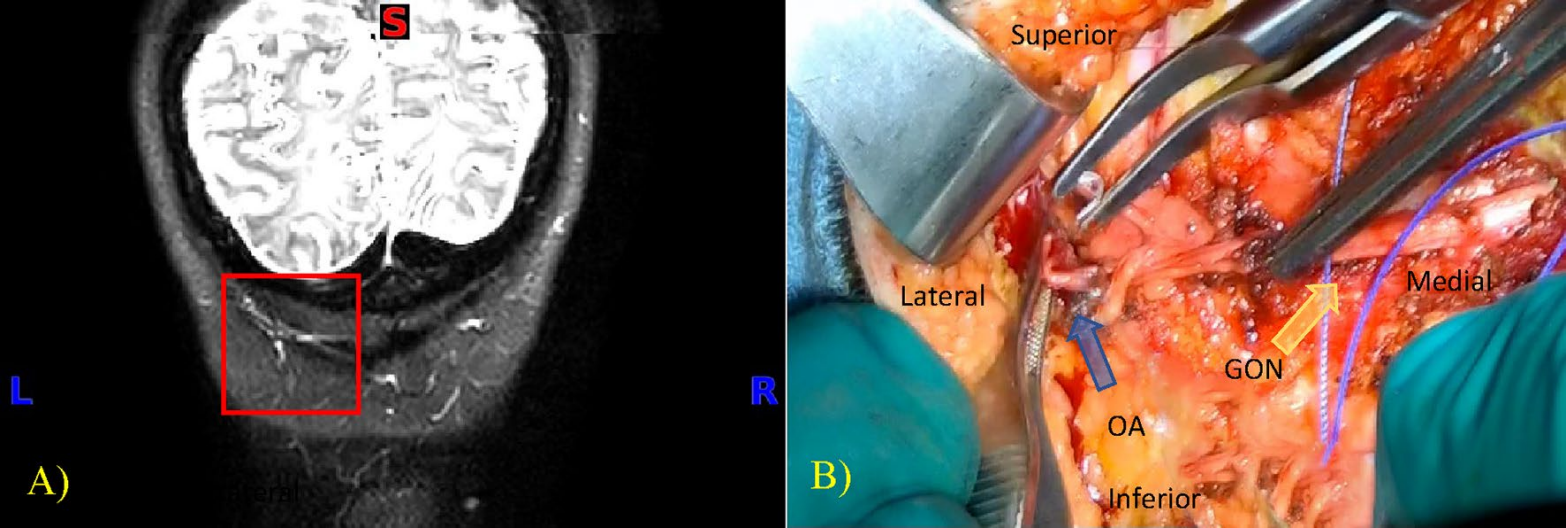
Coronal NerveView MRI tracking the left GON. (A) Two crossing structures with high intensity signals (red box). (B) Intraoperative image showing the GON (yellow dotted lines) and the OA being clipped and separated from the GON.
